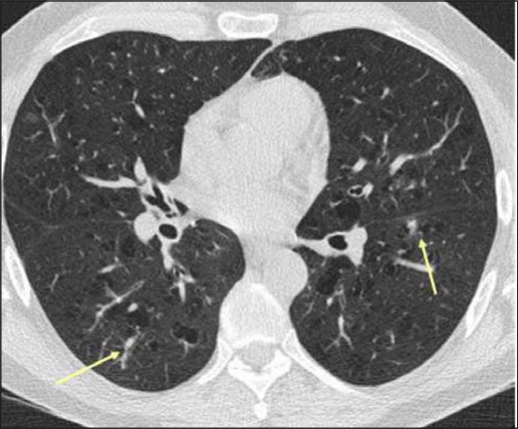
Non-tuberculous mycobacterial infection.* A 50-year-old male and COPD patient had a routine HRCT exam that showed small nodules in both lungs (arrows).
